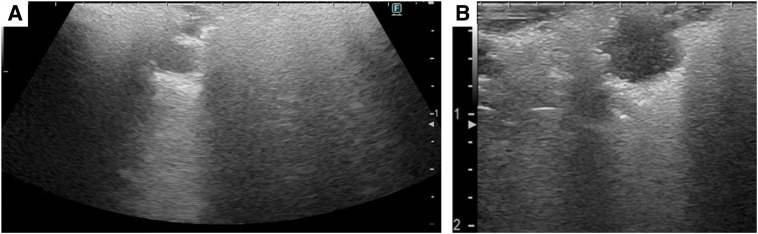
Intraoperative ultrasound imaging. (A) The pulmonary lesion appeared hypoechoic on intraoperative ultrasonography, with the deepest portion exhibiting hyperechoic characteristics. (B) Ultrasound examination of the resected lung confirmed the tumor.
